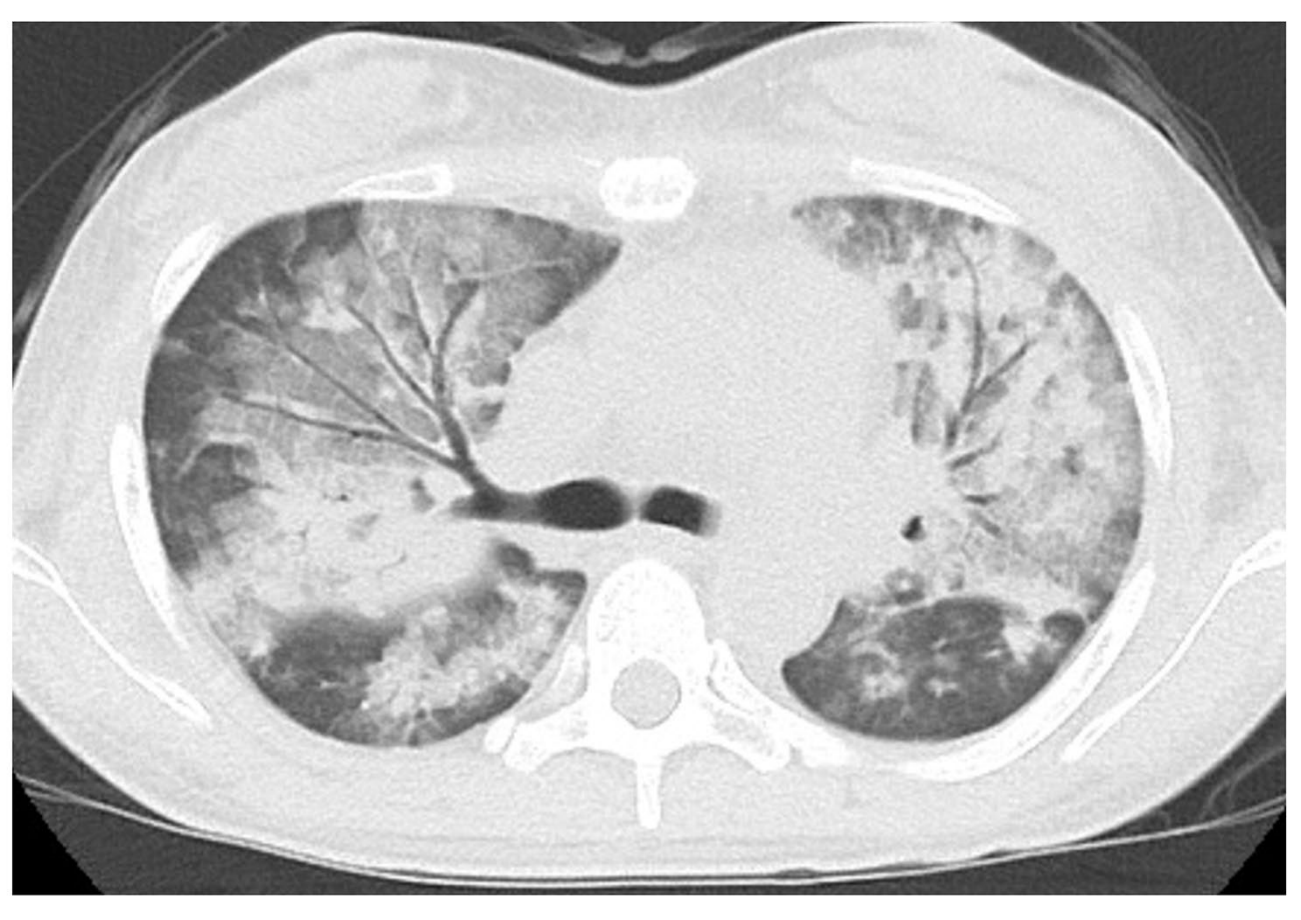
Computed tomography on admission: multiple frosted shadows are seen in the bilateral lung fields
